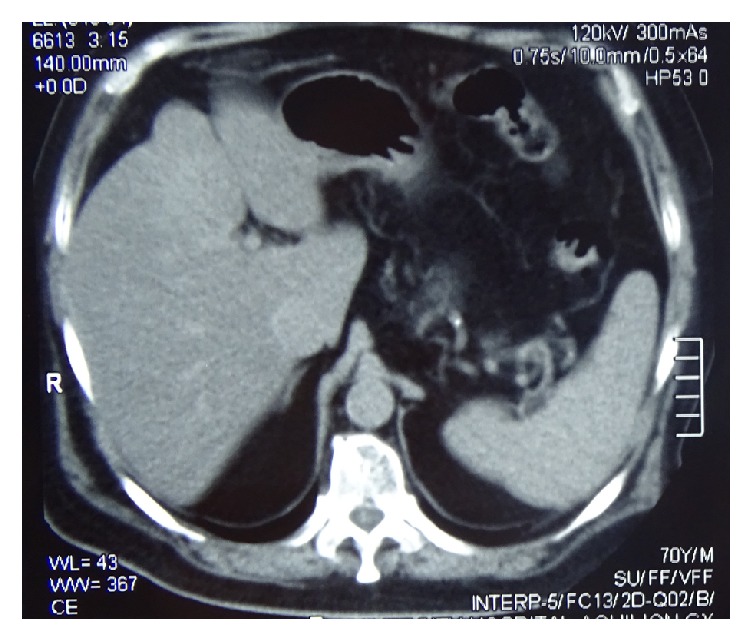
Multi-slice computerized tomography showing no worm even though the ultrasonography showed it.
